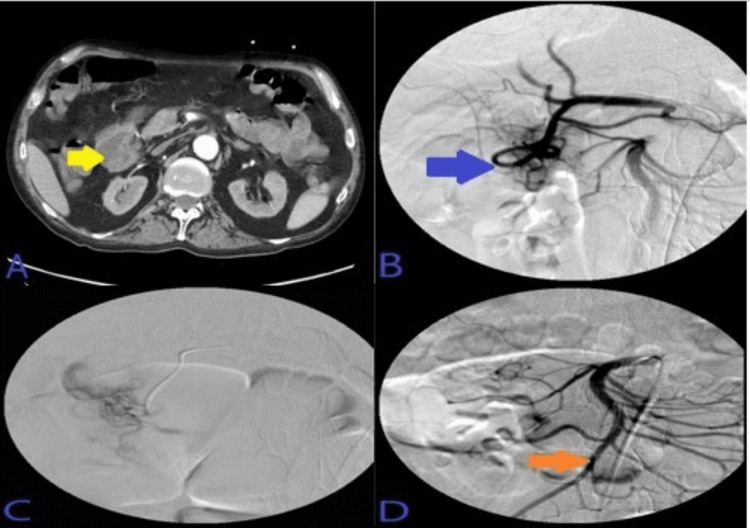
A: CT angiogram of the abdomen showed hyperdense material seen within the second portion of the duodenum compatible with oozing blood seen on endoscopy. B: Selective celiac angiograms demonstrated irregularly tortuous duodenal arterioles as the culprit of bleeding from branch of the GDA with hypertrophic IPDA shunting toward the SMA, therefore no coils were used to avoid non-target migration and bowel ischemia. C: Super-selective GDA angiograms using microcatheter system for embolization using particulate polyvinyl alcohol (PVA) 500 micron particle with full stasis of flow. D: Post-embolization selective SMA angiogram demonstrated patent jejunal, IPDA and ileocecal and colic arteries with no signs of backflow duodenal bleeding. CT: computed tomography; IPDA: inferior pancreaticoduodenal artery; SMA: superior mesenteric artery; GDA: gastroduodenal artery.
